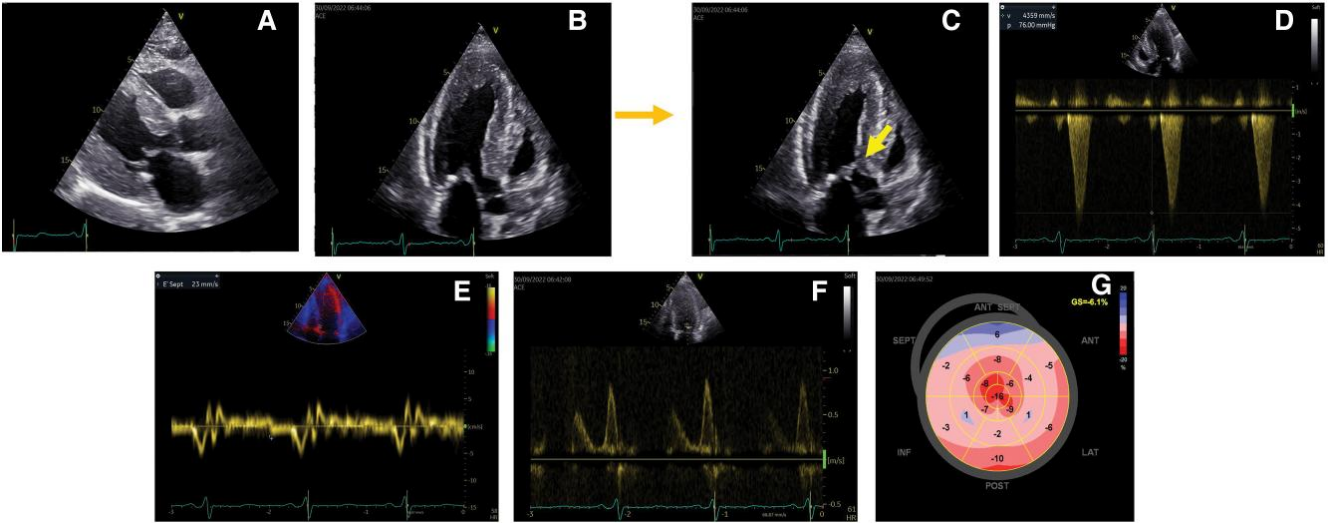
Echocardiographic aspects. (*A*) Parasternal long-axis view showing concentric left ventricular hypertrophy; (*B* and *C*) apical three chambers view showing systolic anterior movement of the mitral leaflet; arrow pointing at contact point with basal septum; (*D*) left ventricular outflow tract gradient 76 mmHg, without provocation manoeuvres; (*E*) low mitral annulus tissue velocities; (*F*) mitral valve left ventricular filing pattern showing *E* < *A*; (*G*) Bull’s-eye plot of global longitudinal strain showing severe longitudinal dysfunction.
